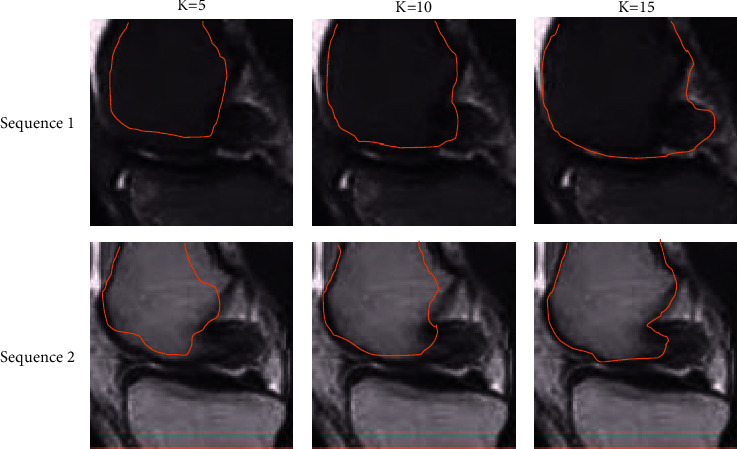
Results of MRI edge detection of knee joint based on intelligent algorithm.
